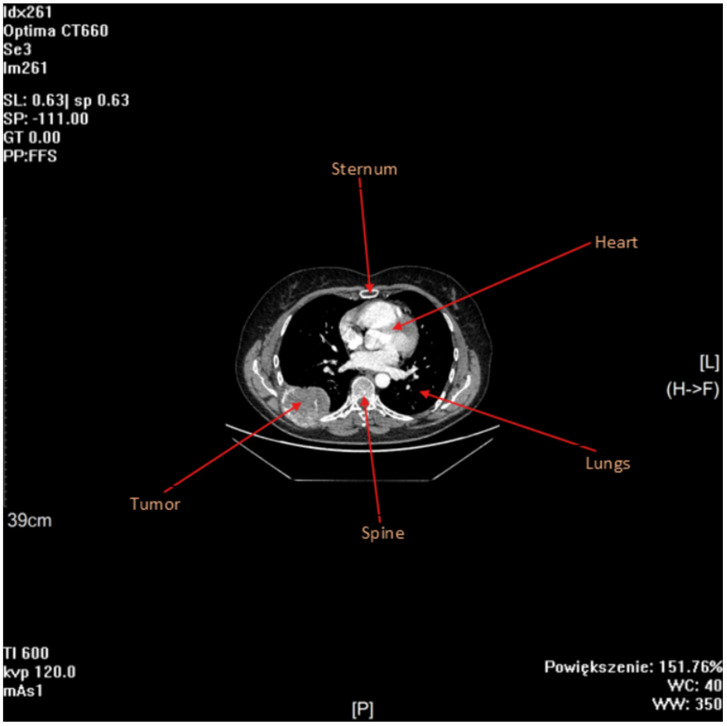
Chest CT scan before tumor resection.
